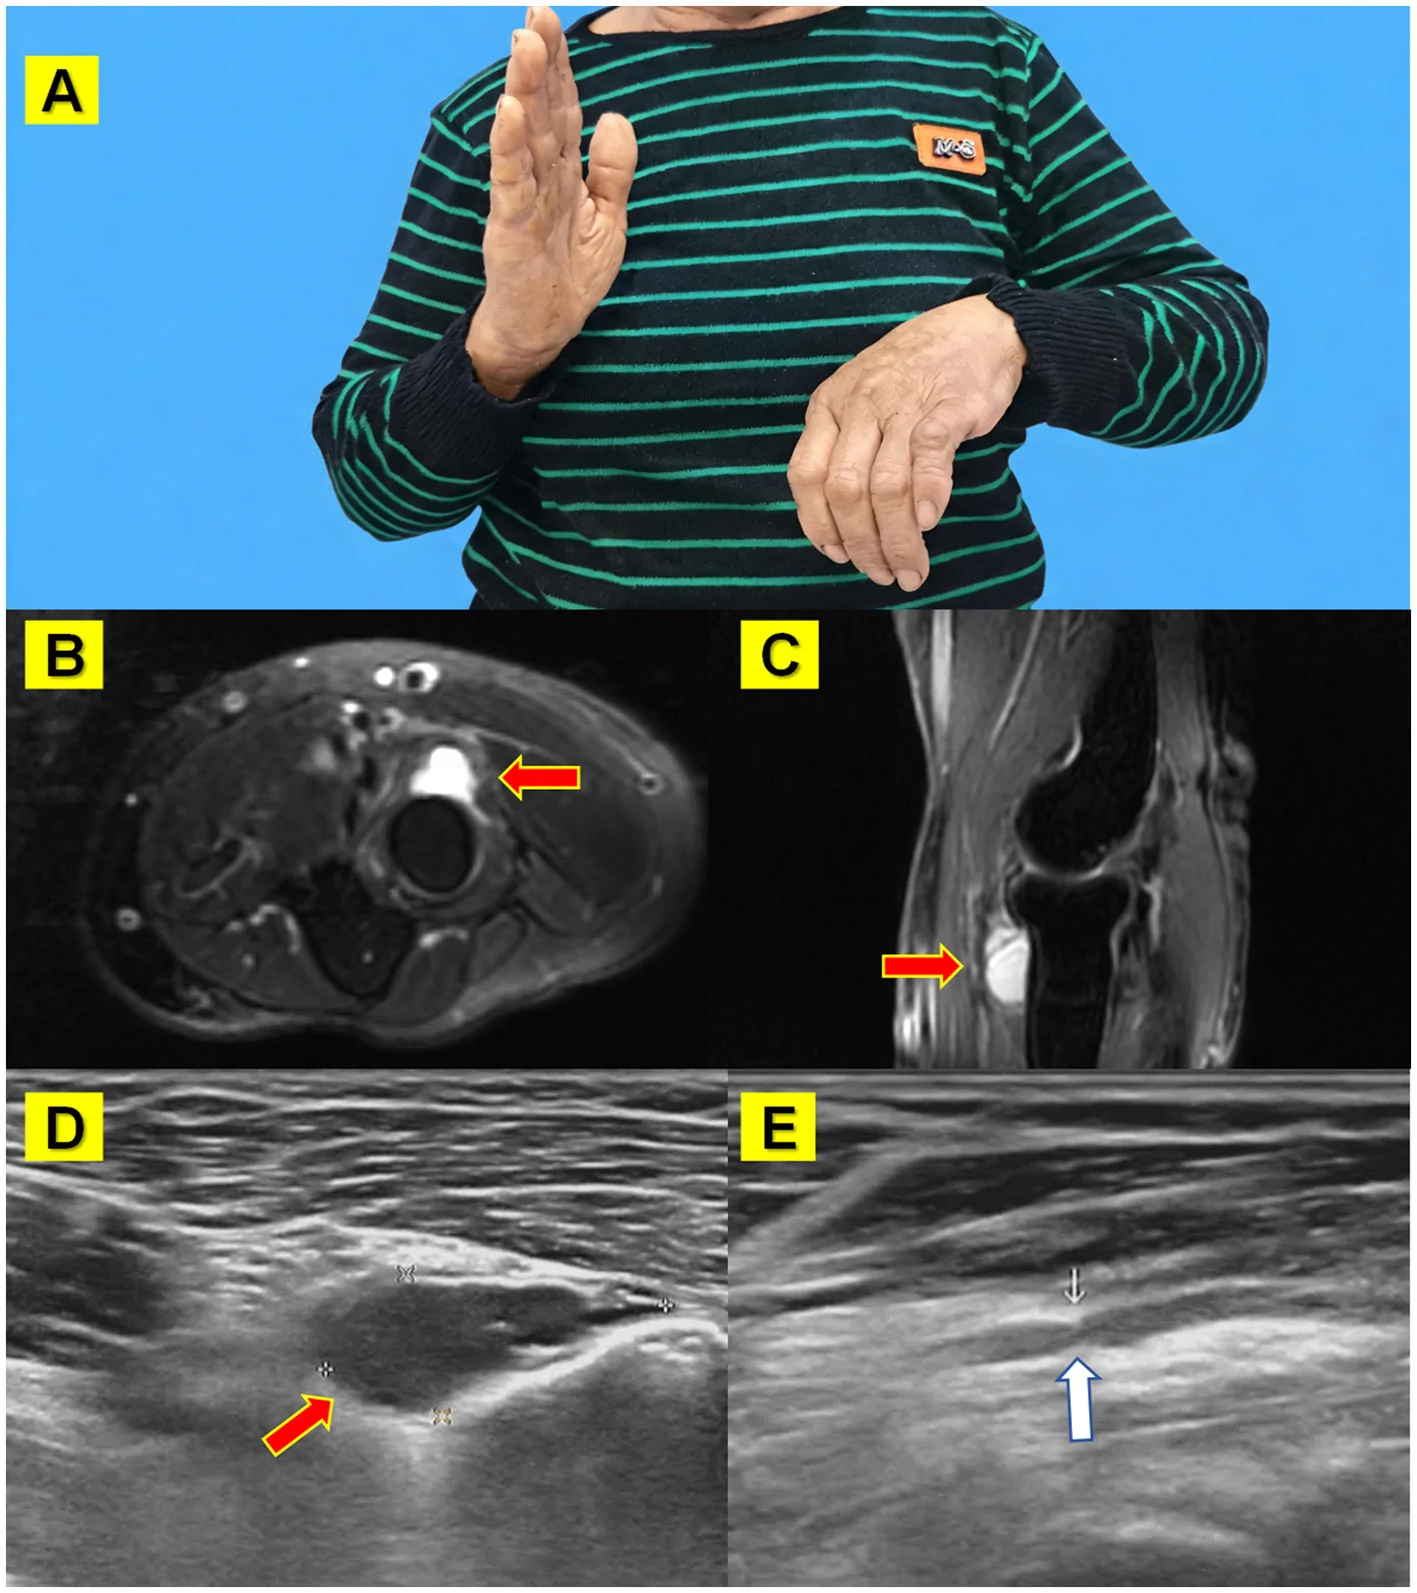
(A) Preoperative posture of the affected limb, demonstrating wrist drop deformity. (B–C) T2-weighted fat-suppressed axial and sagittal MRI images, show a high-signal cystic mass measuring approximately 2.5 cm × 1.5 cm, located in the superficial distal portion of the radial head and the deep proximal portion of the supinator muscle (red arrow). (D) Longitudinal ultrasound section revealed a cyst anterior to the elbow joint, measuring approximately 2.5 cm × 1.5 cm. (red arrow). (E) An hourglass-like constriction was identified at the proximal portion of the deep branch of the radial nerve (white arrow).
